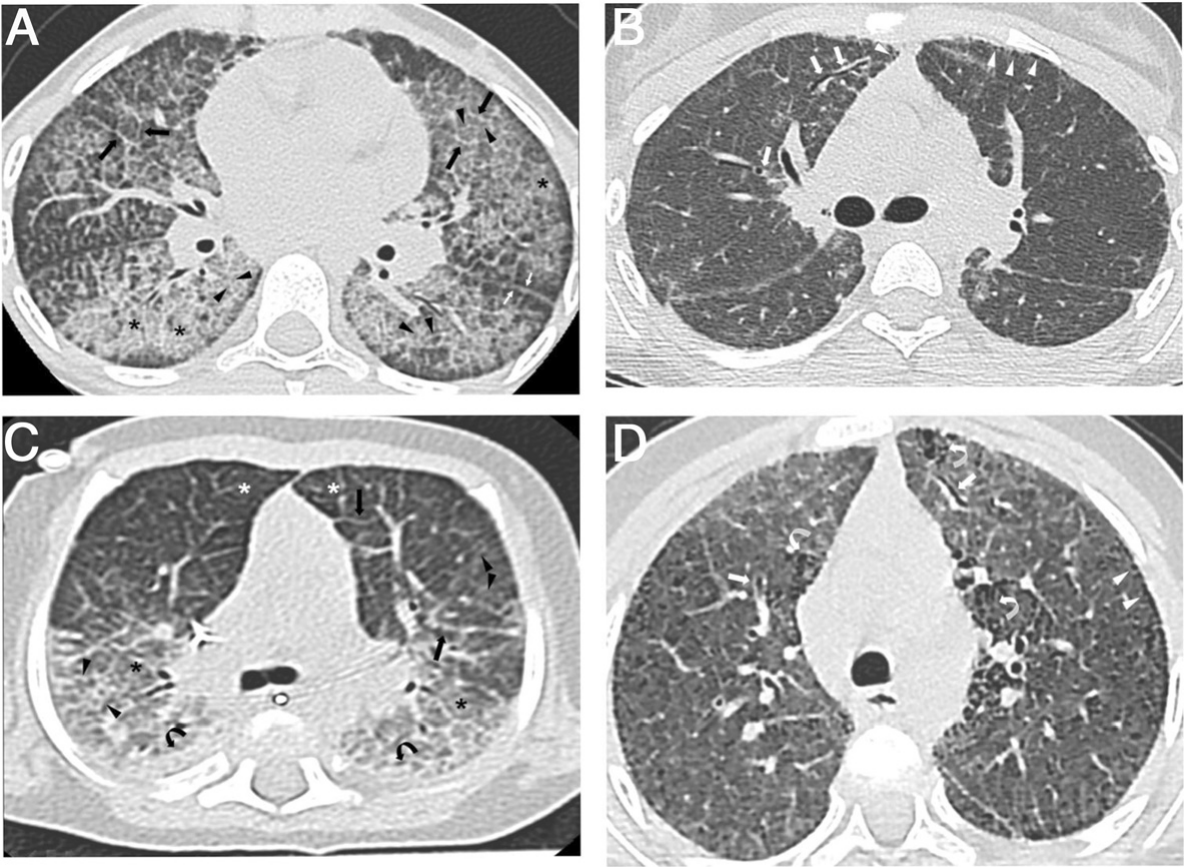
Radiological features. A and B: CT scan from a girl at the ages of 9 (A) and 13 (B) years old. A: Typical aspect of crazy paving pattern on thin axial sections: extensive areas of ground glass opacities (asterisks) superimposed with interlobular septa thickening (black arrows) and intralobular lines (black arrowheads). Irregular thickening of fissures are also noted (white arrows). B: Ground glass opacities, interlobular septa thickening and intralobular lines are less extensive than on the first CT scan while cystic lesions of a few millimetres (white arrowheads) are observed, with a subpleural distribution. Irregular traction bronchiectasis (white arrows) appeared that are initial signs of pulmonary fibrosis. C and D: CT scan of a girl at the ages of 8 months (C) and 5 years old (D). C: This CT scan image shows a symmetric combination of extensive ground-glass opacities (asterisks), intralobular lines (arrowheads) and interlobular septa thickening (black arrows), associated to consolidation (black curved arrows) in posterior areas of the lungs. Note the airspace hyperinflation in the anterior compartment of the lung (white asterisks) responsible of an increasing density gradient from anterior to posterior areas. D: Extensive ground-glass opacities, intralobular lines and interlobular septa thickening are still present. Subpleural cystic lesions (white arrowheads) and signs of fibrosis: honey-combing (white curved arrows) and traction bronchiectasis (white arrows) appeared.
